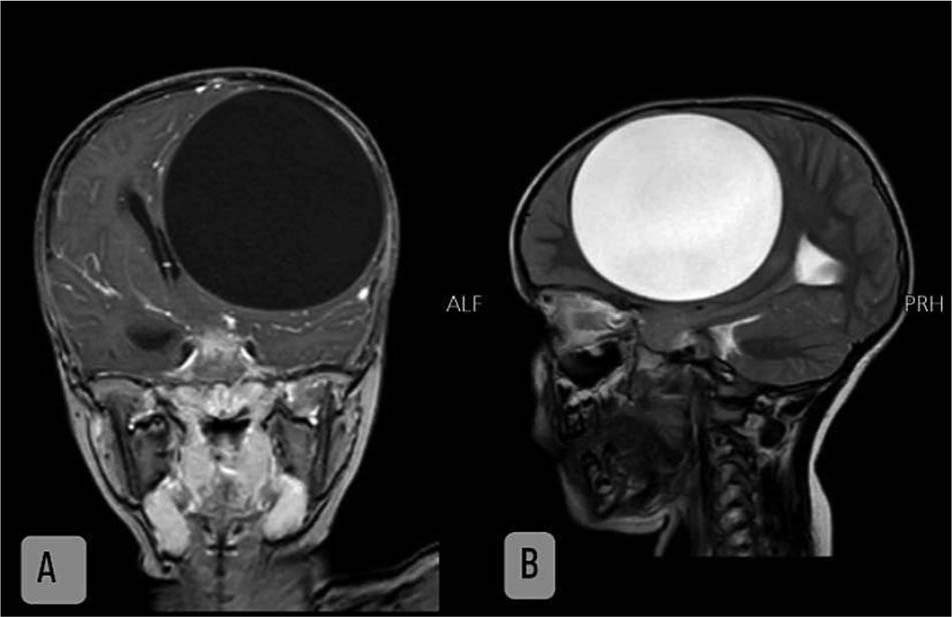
Coronal (A) and sagittal (B) view MRI shows intra-axial cystic lesion measuring 10 × 8 × 9 cm lies at left frontoparietal area, cyst fluid is isointense with CSF.
